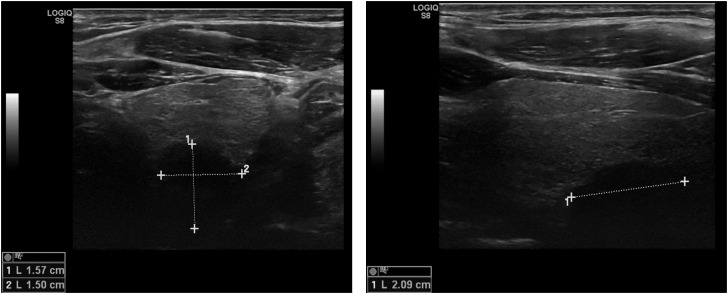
Ultrasound of the thyroid gland examination at first clinical presentation.
